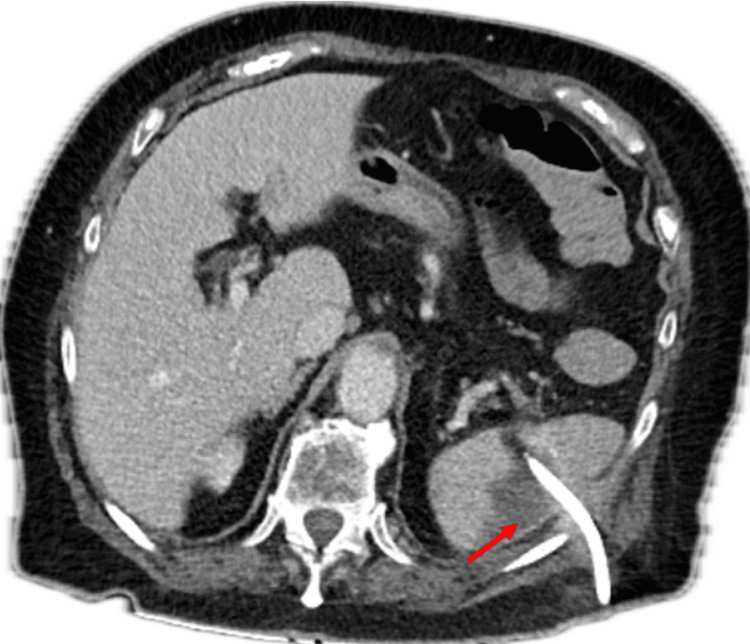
CT post-interventional imaging-guided drainage demonstrating a reduction in collection prior to discharge (red arrow)
